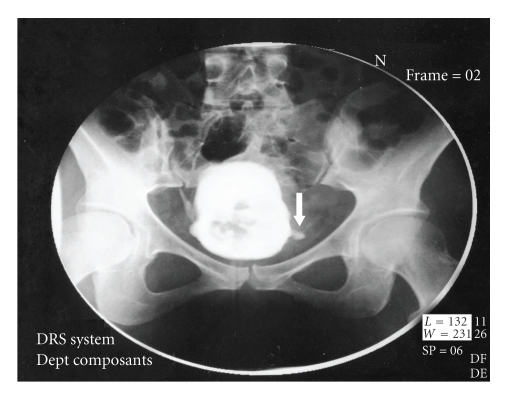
X-ray of bladder: the foreign body penetrate the left wall of bladder.
